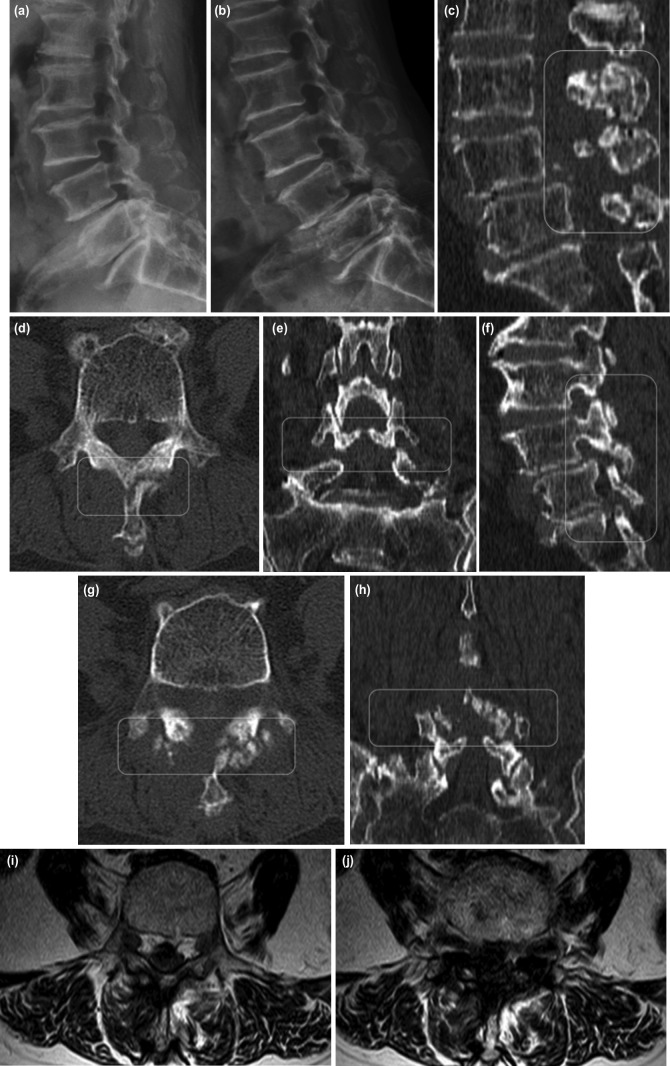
(a) Postoperative lateral radiograph at the time of BE-ULBD (b) and three months follow-up. (c, d, e) MRI and CT imaging for three months after the first surgery (BE-ULBD. Pre-operative CT cut showed a stress fracture of posterior arch at L3, (f, g, h) and L4 (white rounded rectangle). (i) Pre-operative T2 weighted MRI axial cut L4 spondylolytic spondylolisthesis, severe herniated nucleus pulposus and L3 and L4 posterior arch fracture. (j) Axial cut at L4-L5 level.
